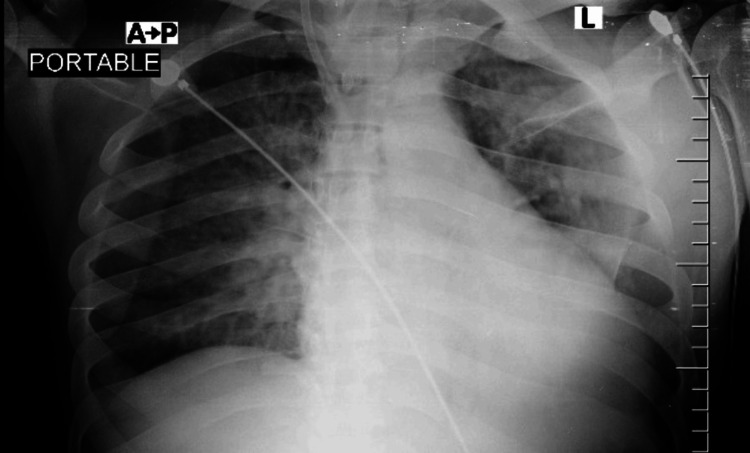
Initial chest X-ray reveals ground-glass changes.
